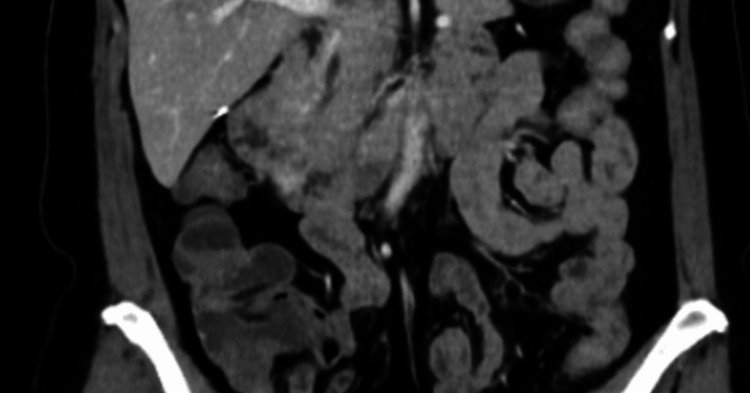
CT abdomen at the three-year follow-up showed no evidence of recurrence
